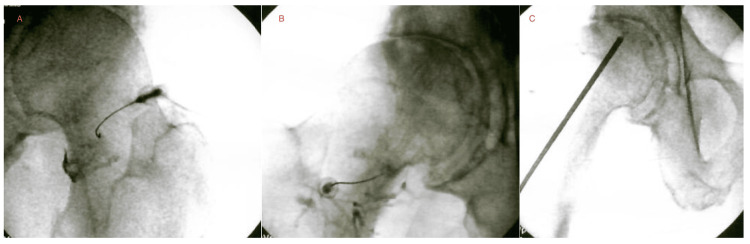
Patient 5 - bilateral hip BMC injections Fluoroscopy-guided bilateral intra-articular injections (A, B) and right hip intraosseous BMC injection (C). X-ray of the hips indicated a Tönnis score of 3. At follow-up, the patient reported VAS improvement from 9 to 2 and 70% SANE improvement in function after the second treatment. BMC, bone marrow concentrate; SANE, single assessment numeric evaluation
